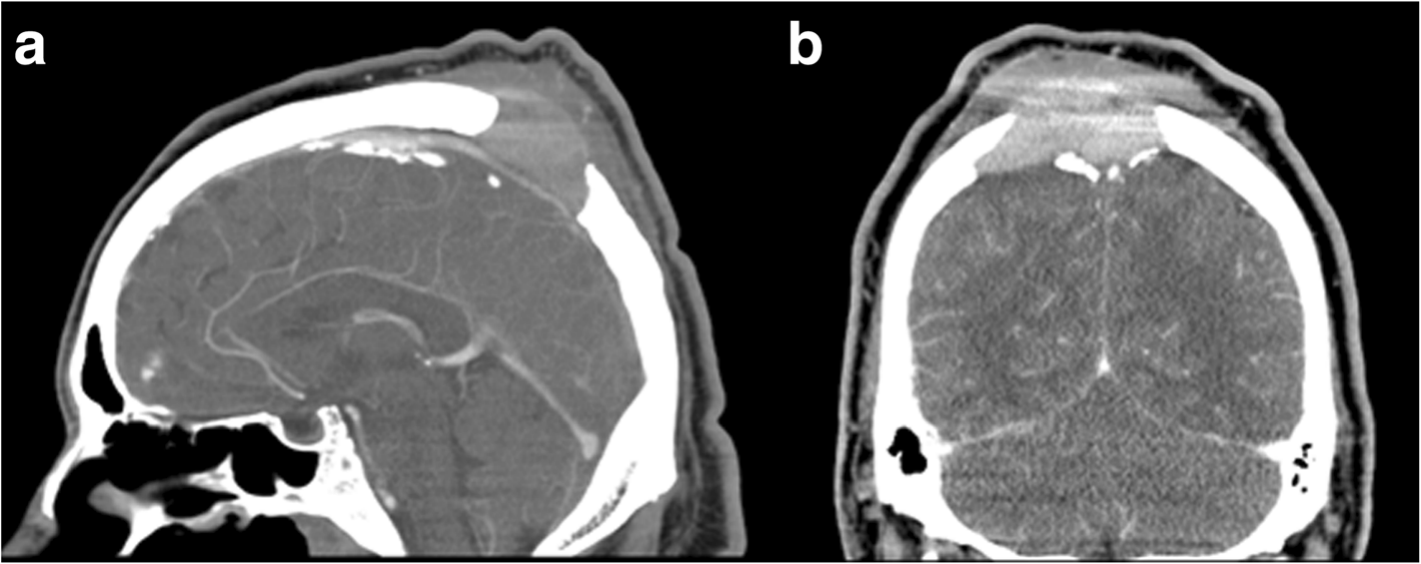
Head computed tomography scan. (a) Sagittal and (b) frontal views of a contrast-enhanced cranial computed tomography scan. The intense bone destruction above the sagittal sinus can be seen. No brain infiltration seems to be present.
